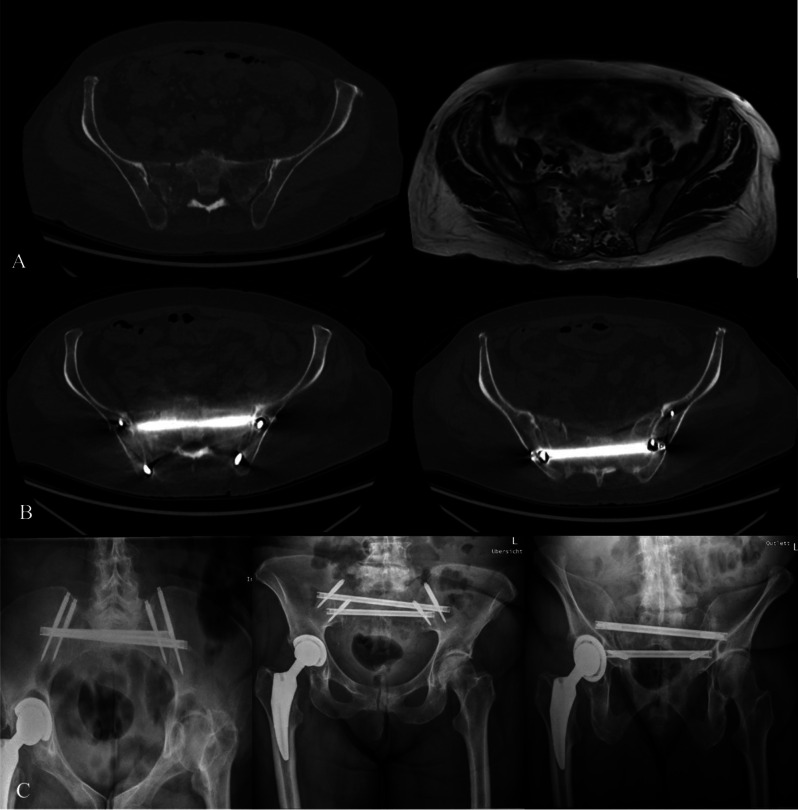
Patient 1 A- Axial CT and MRI scan presenting pathological sacral fracture, B- postoperative CT, C- X- ray postoperative
